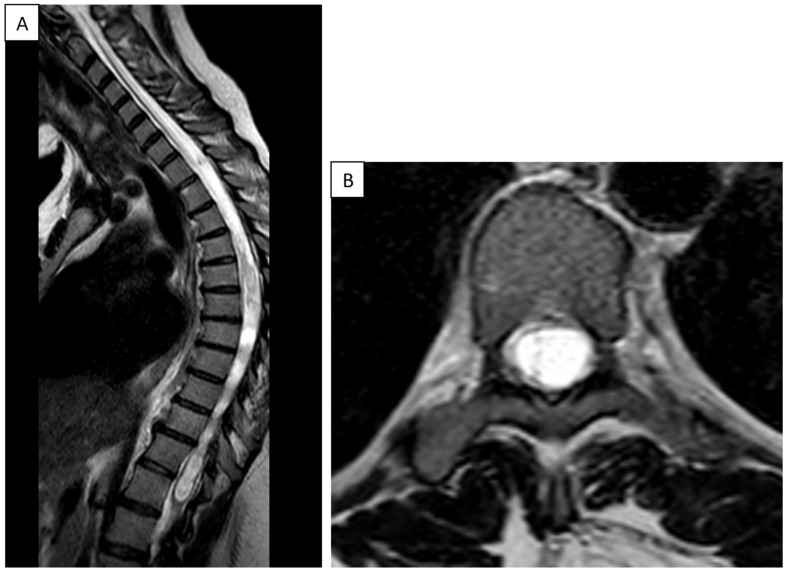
MRI of the thoracic spine in a patient with syringomyelia. (A) Sagittal T2-weighted image demonstrating an elongated, hyperintense intramedullary cavity consistent with a syrinx extending along the thoracic spinal cord. (B) Axial T2-weighted image showing a well-defined syrinx centrally located within the spinal cord, causing expansion of the cord parenchyma.
